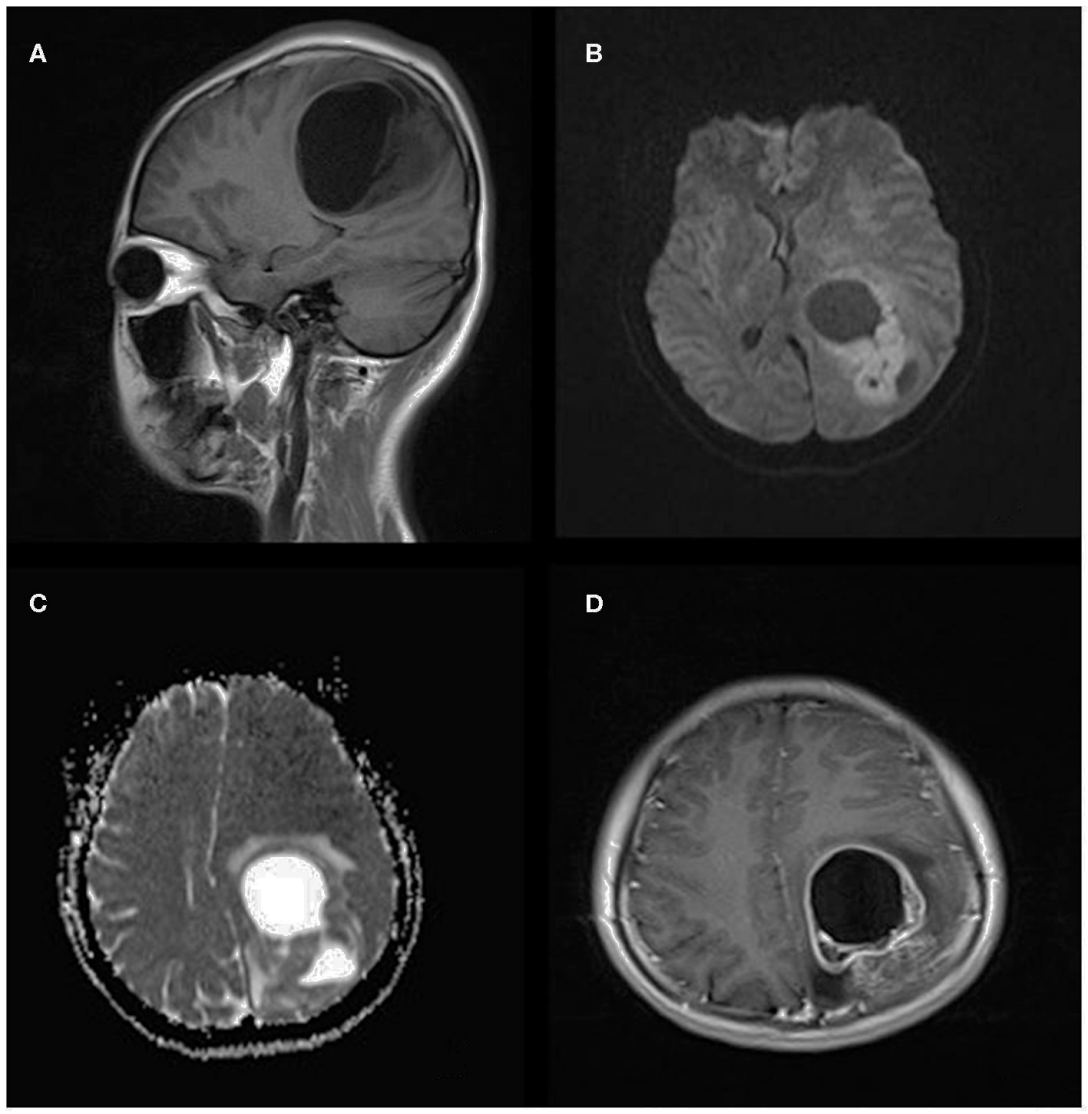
A 21-year-old woman with supratentorial intraparenchymal anaplastic ependymoma in the left parietal lobe on (A) T1-weighted image, (B) diffusion-weighted images, (C) apparent diffusion coefficient, and (D) contrast T1-weighted image. The tumor appears as heterogeneous signal intensity, large cysts, reduced diffusion within the soft tissue component, and mild peritumoral edema.
